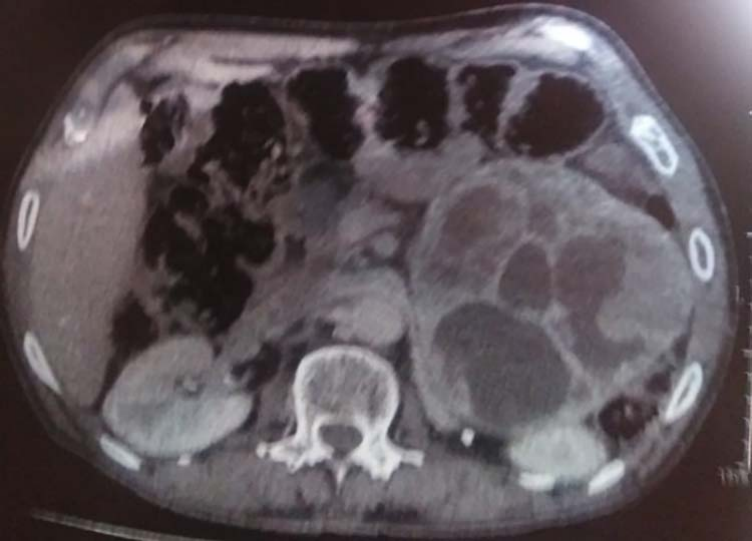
CT scan image showing left retroperitoneal cyst.
